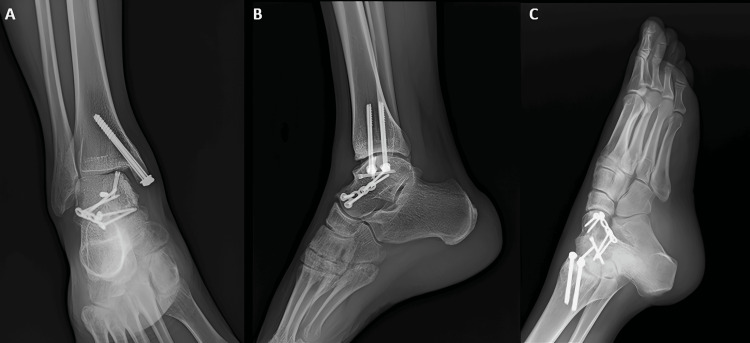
X-ray images of the right ankle. The images show (A) anteroposterior view on follow-up showing united talus fracture with no signs of avascular necrosis of talus and implant in position, (B) lateral view on follow-up showing united talus fracture with the implant in position, and (C) Canale and Kelly's view of the right ankle and foot on follow-up showing a united talus fracture and implant in position.
